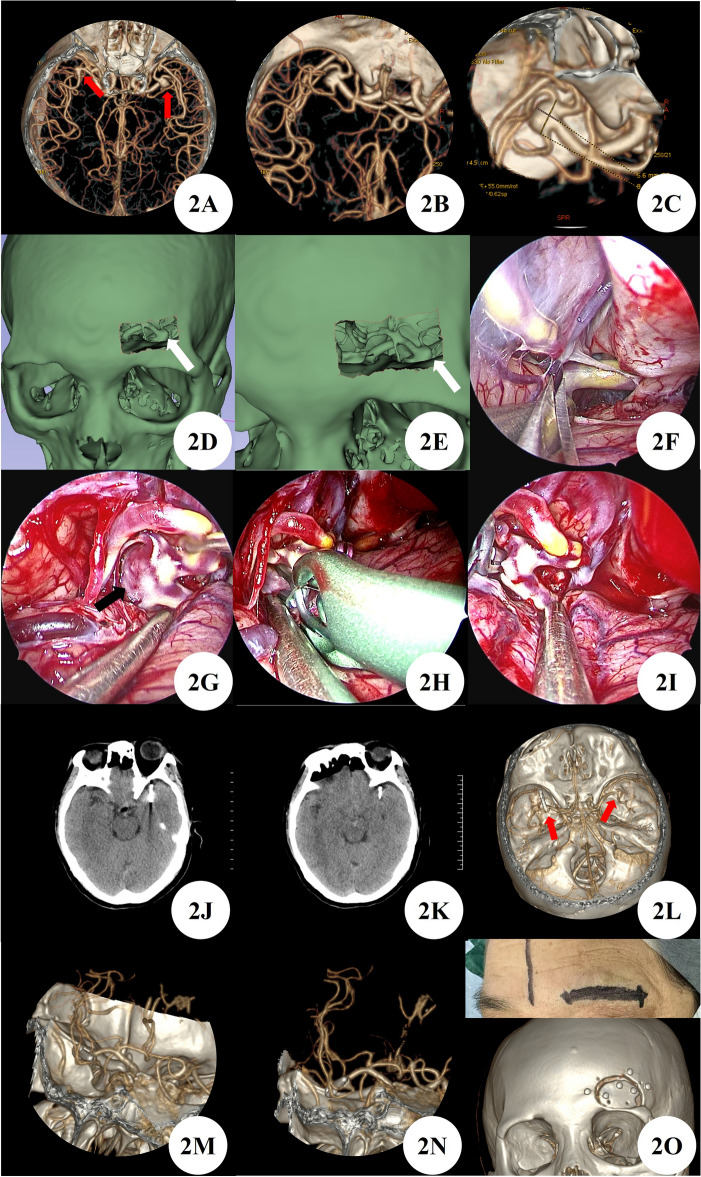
(A–C) Preoperative CTA showed bilateral middle cerebral artery bifurcation aneurysms and left middle cerebral bifurcation aneurysms that require priority treatment (red arrow). (D,E) Feasibility evaluation of supraorbital eyebrow arch keyhole surgery using 3D Slicer reconstruction virtual reality technology (white arrow). (F–I) Neuroendoscopic clipping of left middle cerebral artery bifurcation aneurysm. (J,K) Postoperative CT showed aneurysm clipped. (L–N) After clipping the aneurysm at the bifurcation of the left middle cerebral artery in postoperative CTA, no stenosis of the parent artery or branch was observed, and an untreated aneurysm at the bifurcation of the right middle cerebral artery was found (red arrow). (O) Surgical incision and bone window.
